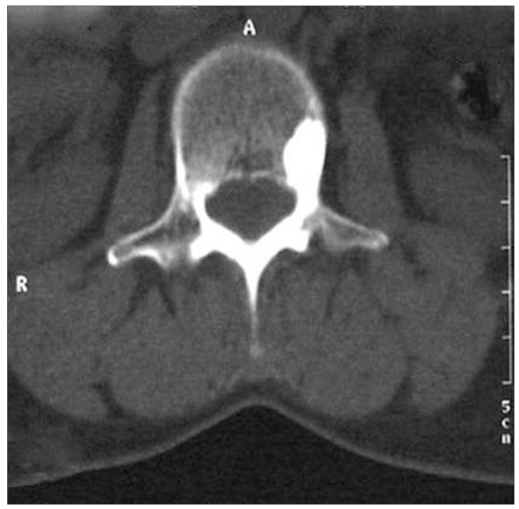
Further evaluation with CT showing hyper dense sclerotic lesion diagnosing benign osteoma
